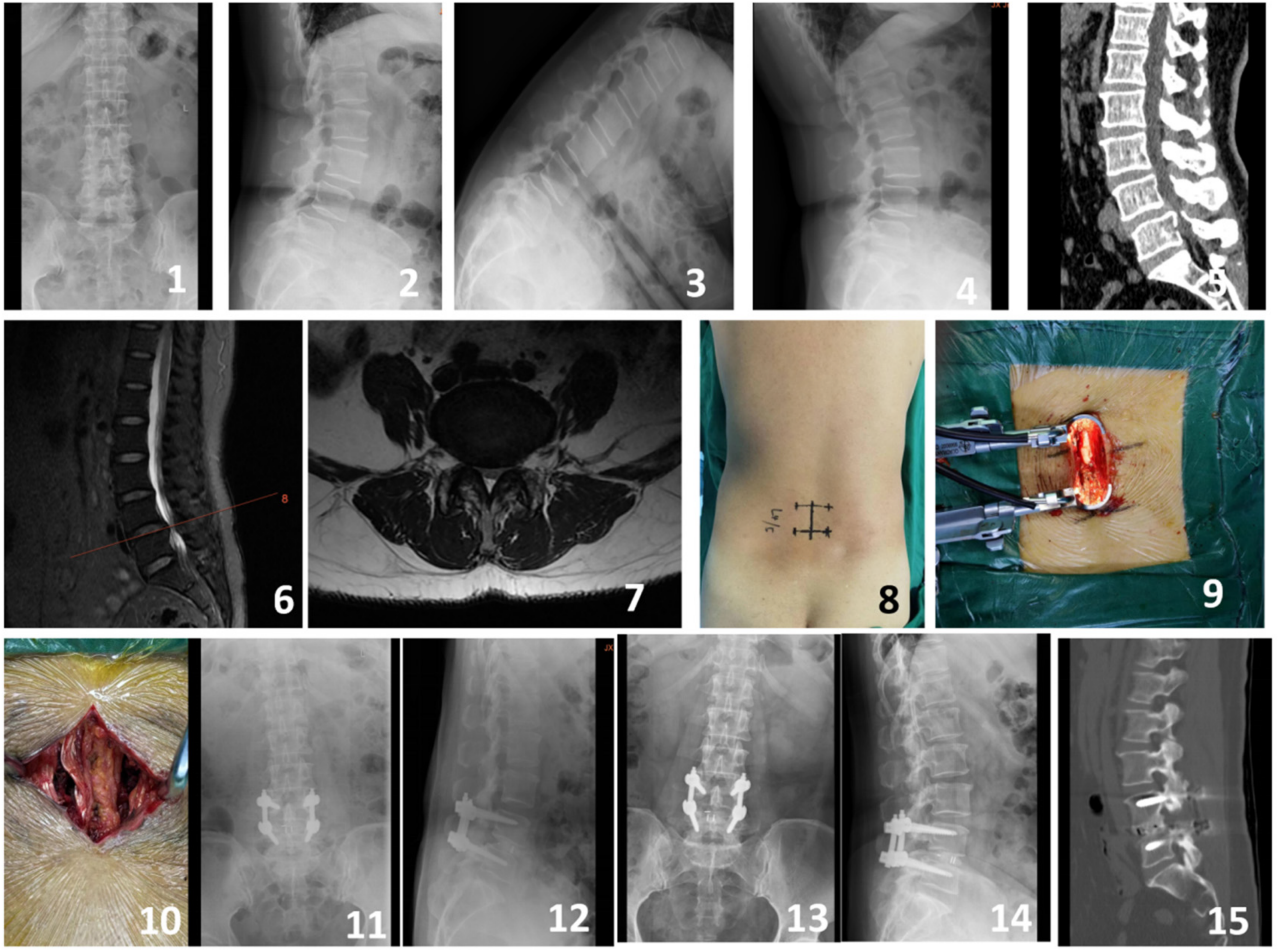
A 64-year-old male presented with intermittent claudication and numbness in the right lower limb for 2 years. Preoperative imaging included lateral, anteroposterior, and dynamic flexion-extension lumbar radiographs (Figures 1-4), preoperative CT scan (Figure 5), and preoperative MRI (Figures 6-7). Intraoperative images captured the process of the modified single-incision surgery (Figures 8-10). Postoperative imaging at 3 days following surgery included lateral and anteroposterior lumbar radiographs (Figures 11-12). Follow-up imaging at 6 months postoperatively included lateral and anteroposterior lumbar radiographs (Figures 13-14). A CT scan at 12 months postoperatively showed good bony fusion (Figure 15).
